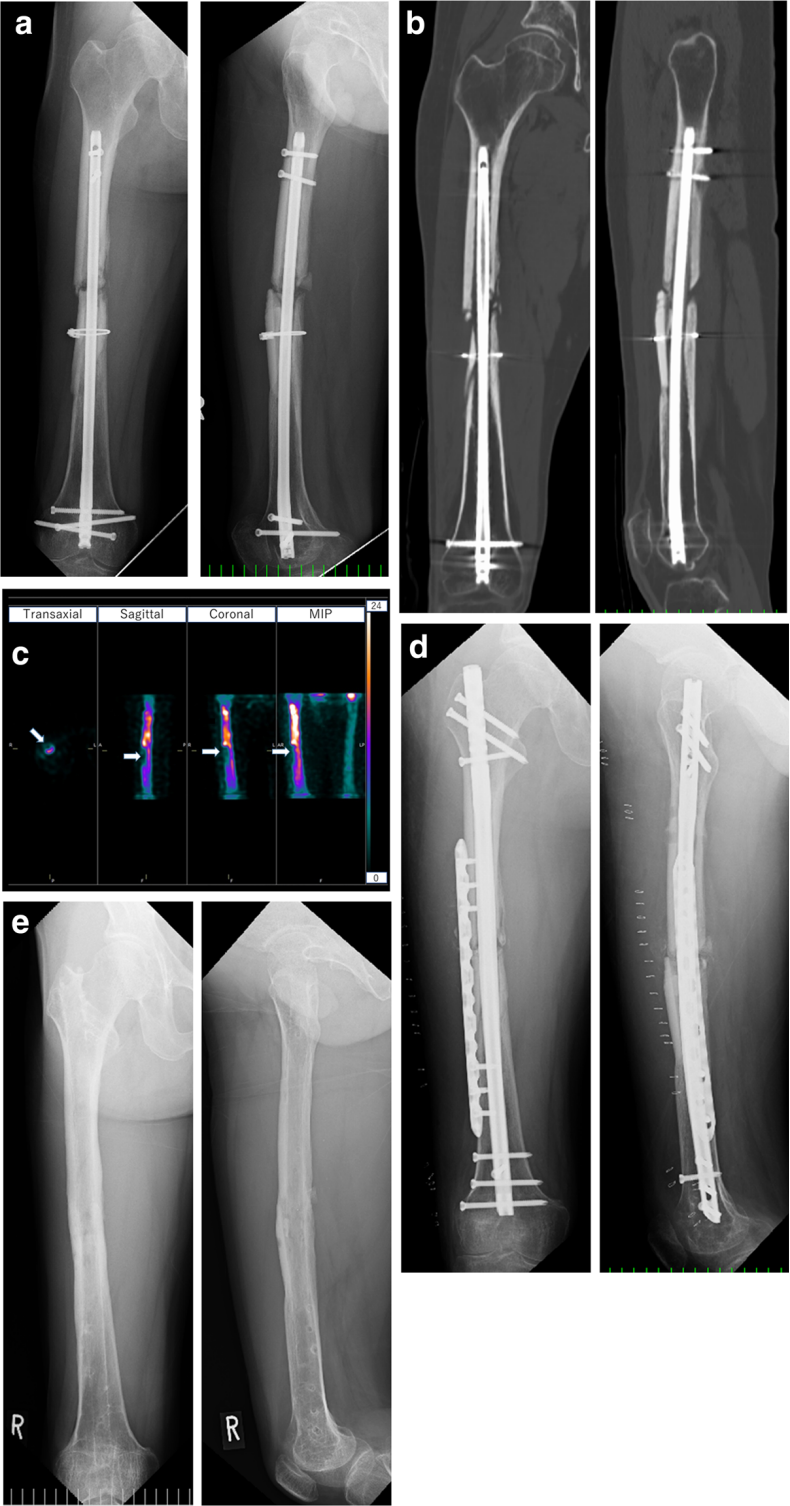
Case 19. A 38-year-old man with noninfected nonunion of the femur. a X-ray image at the time of admission to our hospital. b CT images from the same period. A relatively large bone gap was found around the nonunion site. c Quantitative evaluation by the GI-BONE software. The third bone fragment shows low uptake (white arrow). MIP, maximum intensity projection. d X-ray image immediately after nonunion surgery with augmentative plating, nail exchange, and autologous bone grafting. e X-ray image 6 months postoperatively showing that bone union was achieved. All implants were removed
